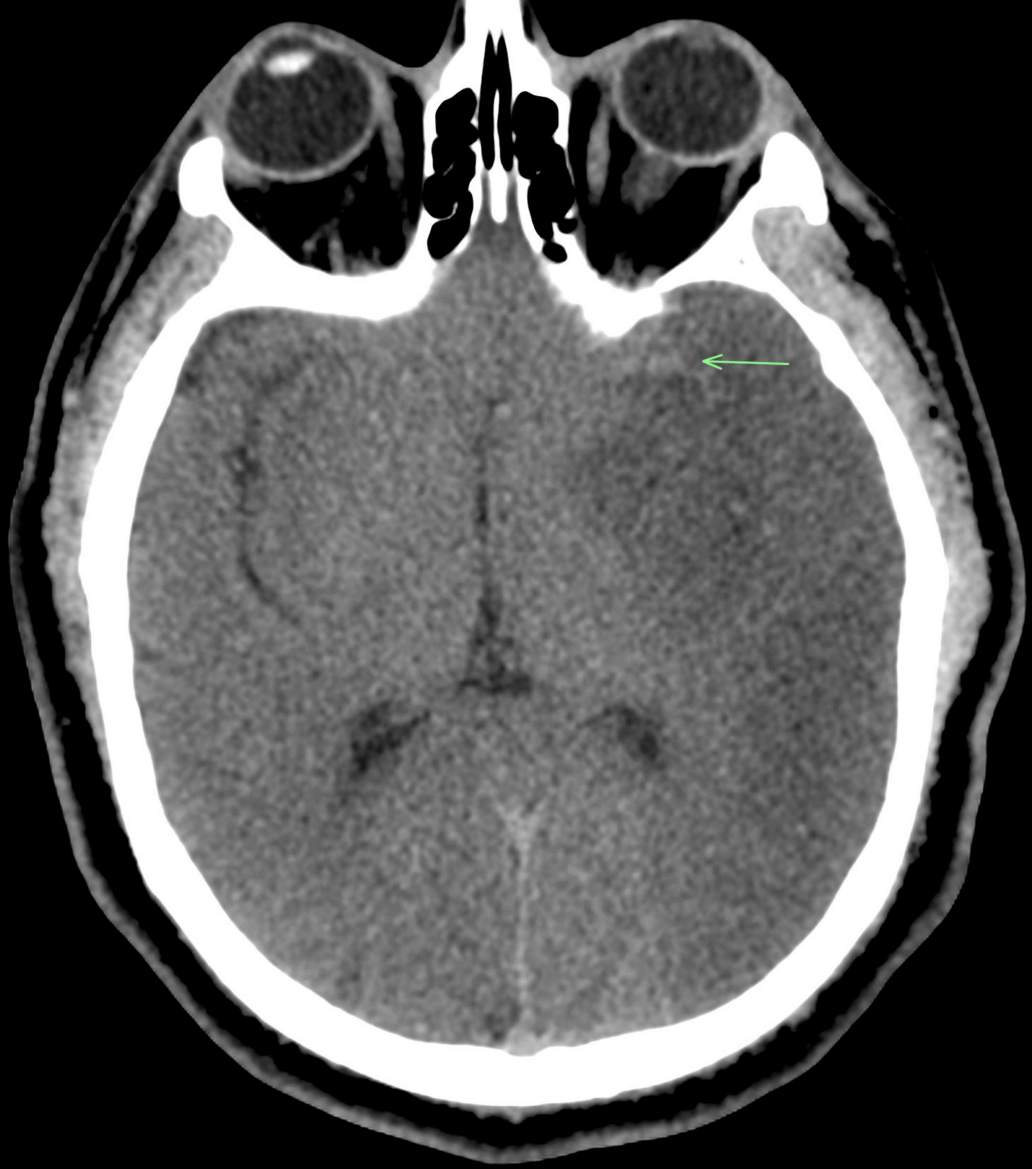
Computed tomography (CT) of the head showing left MCA occlusion and evolving infarct MCA: middle cerebral artery
